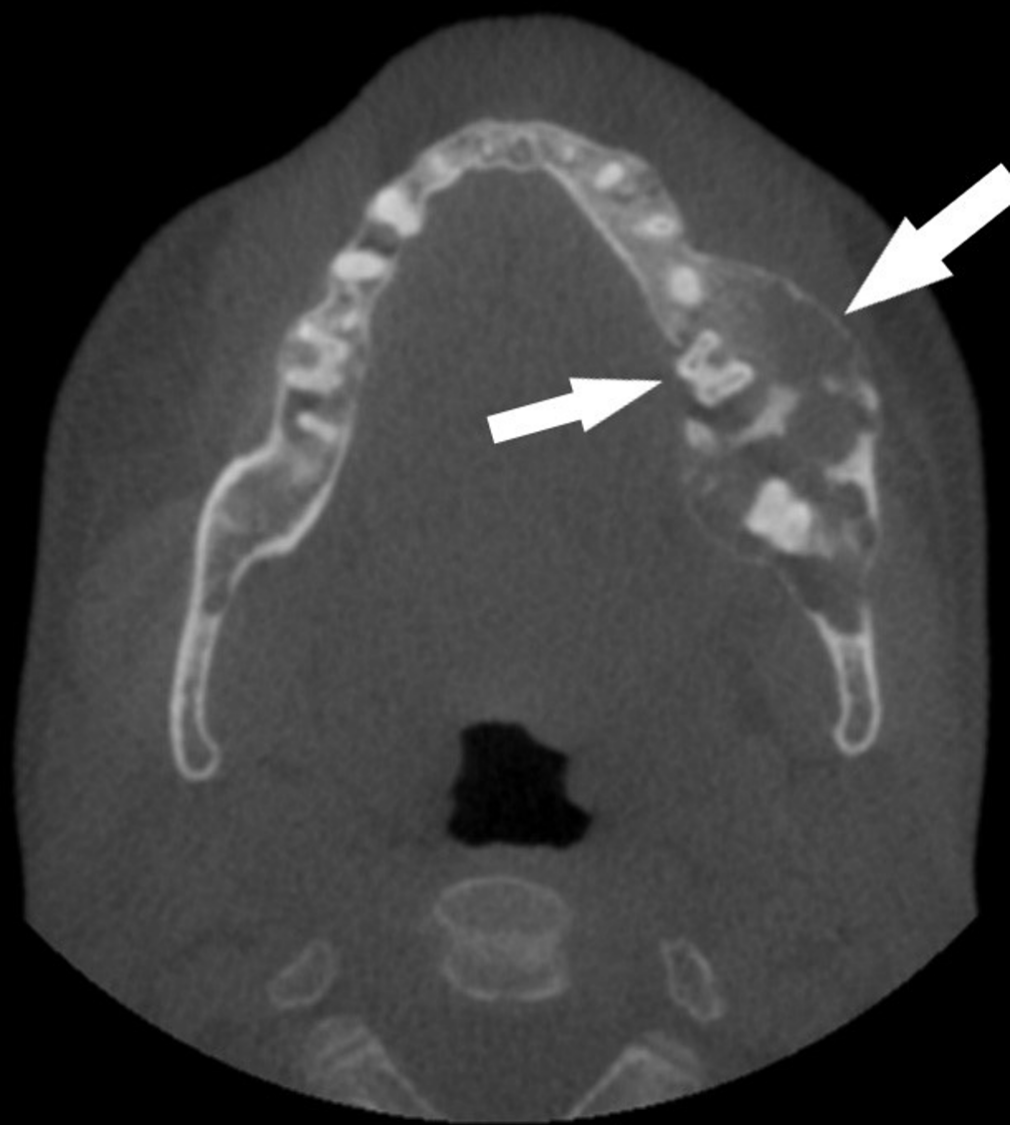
Axially reconstructed CBCT of the jaws depicting buccolingual enlargement and disruption of the lingual cortical plate. CBCT, cone beam CT.
